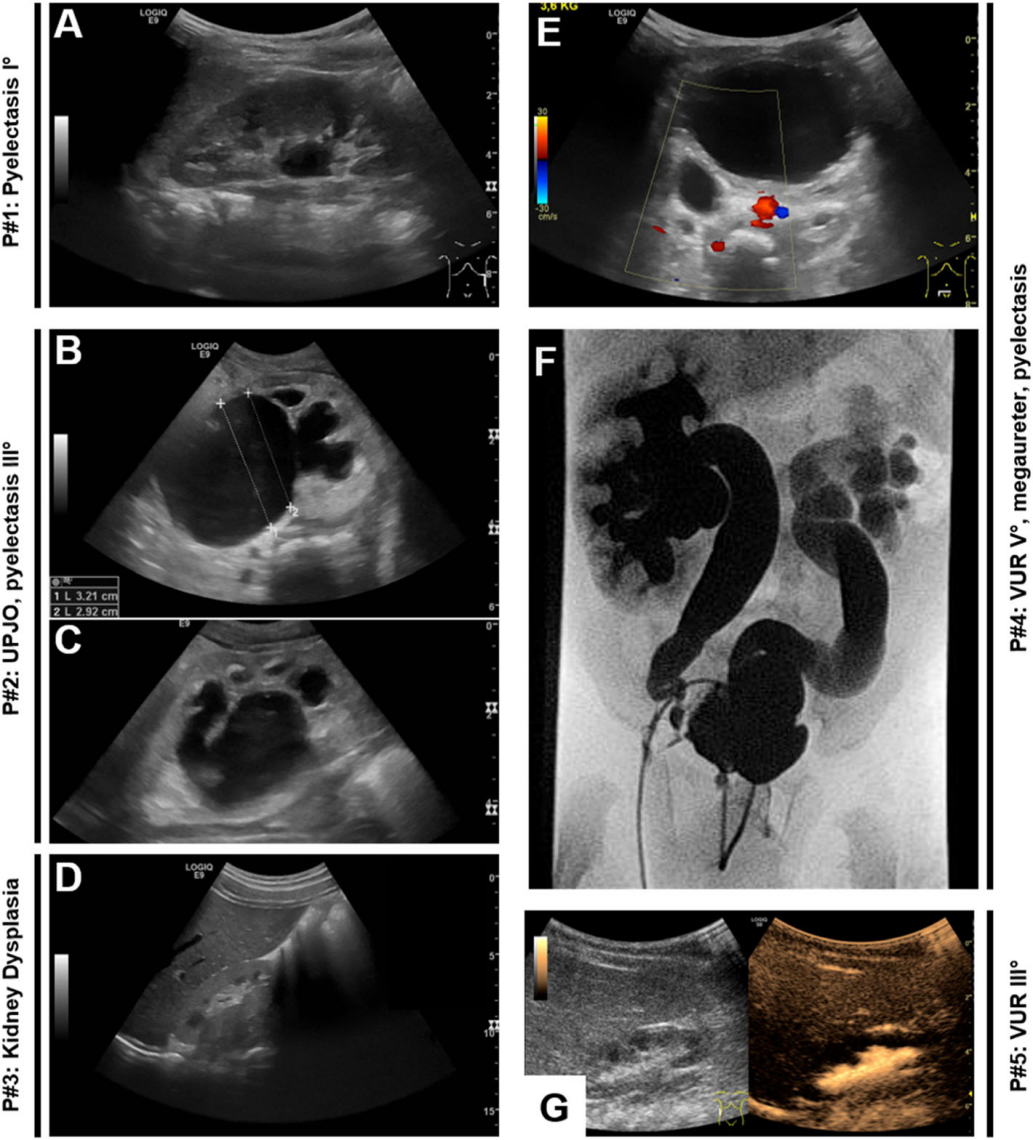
Imaging studies in patients with different CAKUT. (a) Mild dilatation of the kidney pelvis (11 mm); (b and c) severe dilatation of the kidney pelvis (290 mm); and calyxes due to ureteropelvic junction obstruction (UPJO). (d) Small and hyperechogenic right kidney without corticomedullary differentiation suggesting kidney dysplasia; (e) Dilatation of the right ureter retrovesically; (f) voiding cysturetrography (VCUG) demonstrating bilateral VUR V° in the filling phase in the same patient as in e (International Reflux Study Committee (1981)). Contrast agent was administered using a transurethral catheter. (g) Contrast-enhanced uro-sonography in a patient with VUR III°. Contrast agent is detectable in the right kidney pelvis
